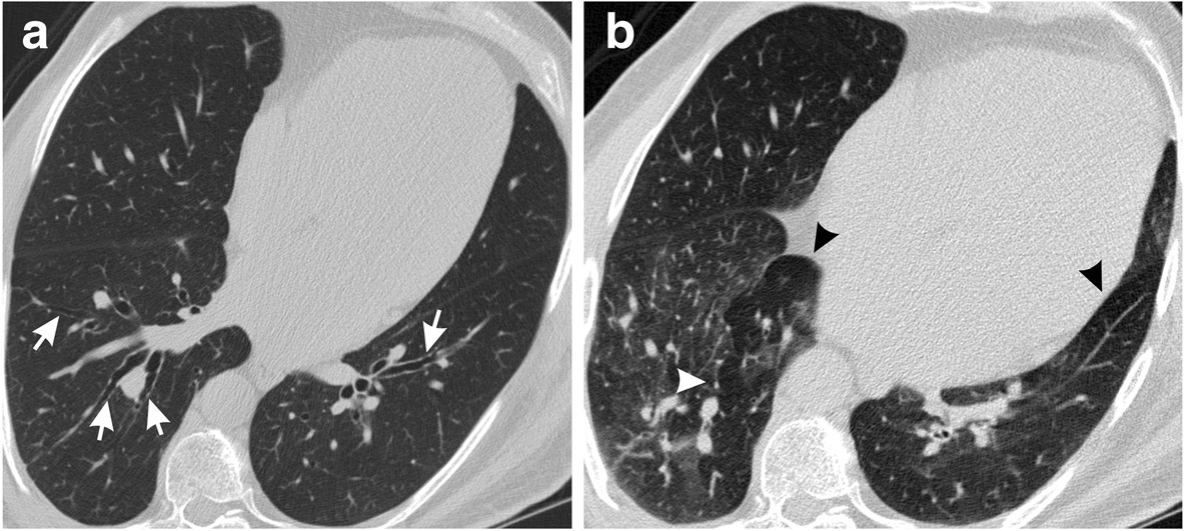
Bronchiectasis and air trapping in a 56-year-old man with acromegaly who had an RV value of 126% of predicted and a Raw value of 5.23 cm H_2_O/L/s. (a) Inspiratory, transverse, thin-section CT scan obtained through the lower lung zone shows bronchiectasis (arrows). (b) Expiratory, transverse, thin-section CT scan obtained through the lower lung zone reveals diffuse air trapping (arrowheads).
